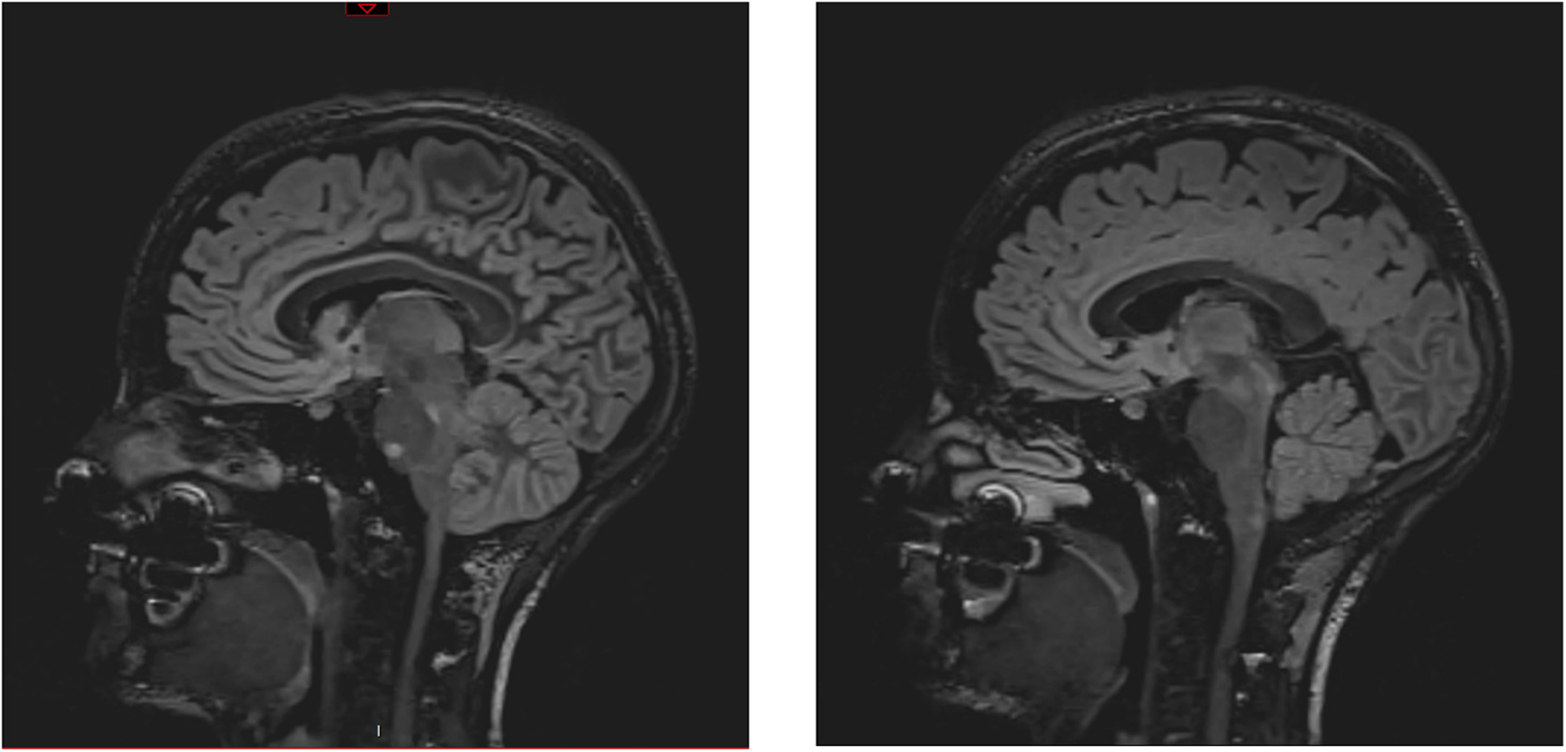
Cerebral magnetic resonance imaging (T2-flair) performed at day one showing three demyelinating in the brainstem.
